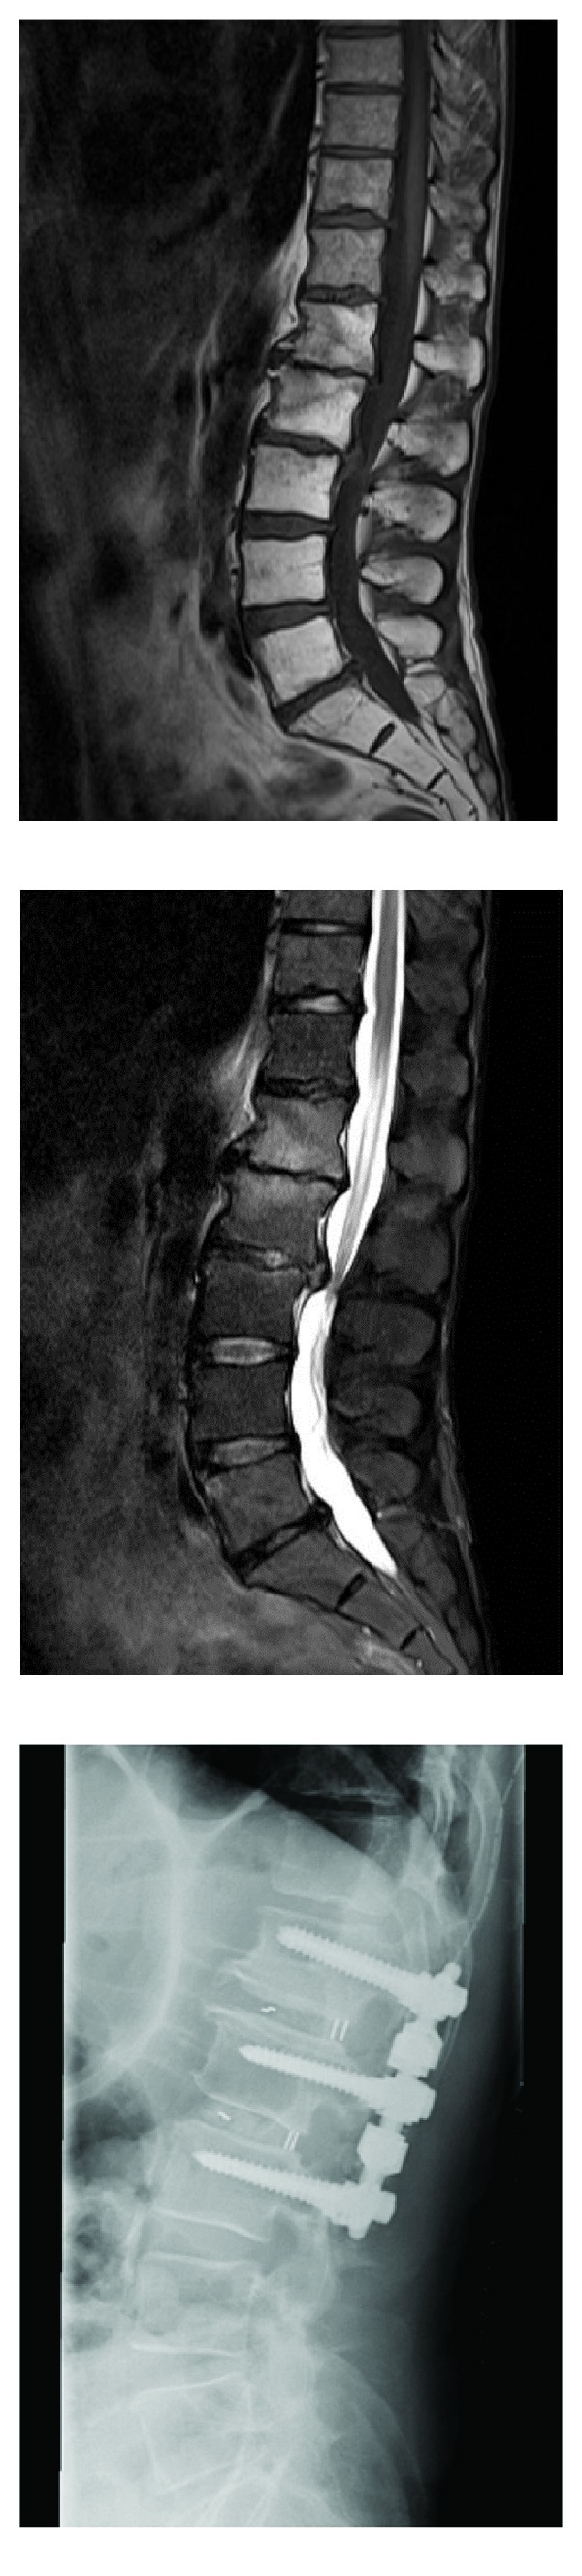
Left: MRI of 55-year-old man with left back and leg pain. Right: immediately postoperative X-ray film.
